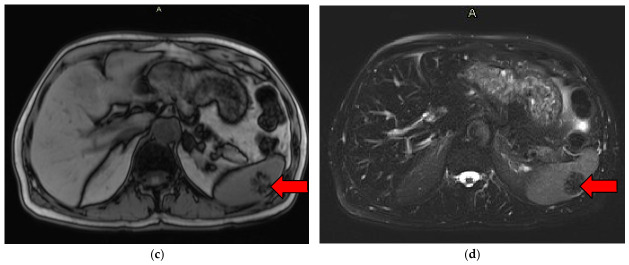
68-year-old male with an incidental splenic lesion. (a) Ultrasound shows a hypo-echoic splenic lesion with ill-defined margins. (b) On portal venous phase contrast-enhanced CT, the lesion is ill-defined, heterogeneous, and hypoattenuating. (c) On an axial T1 out-of-phase imaging, the lesion is well circumscribed with heterogeneous signal intensity and internal radiating septations forming a spoke wheel configuration. (d) On an axial T2-weighted image, the splenic lesion demonstrates low signal intensity with hypo-intense septa, forming a spoke wheel morphology.
